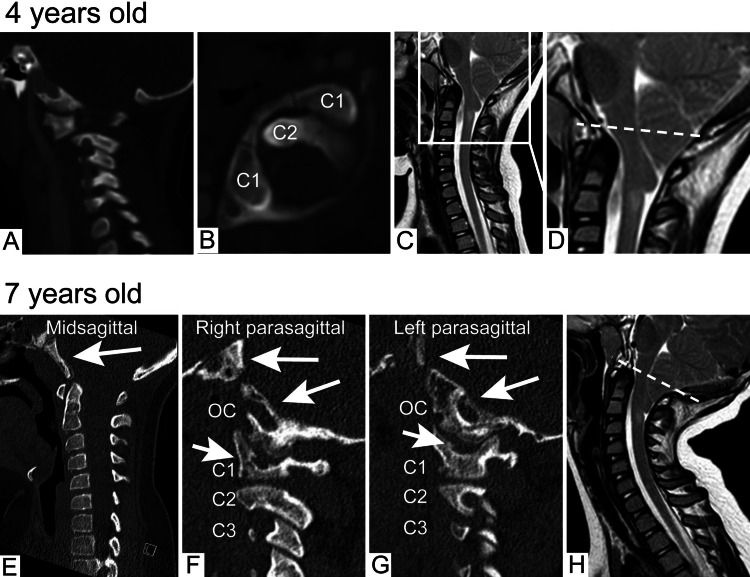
Follow-up imaging when the patient was 10 years old. Midsagittal (A) and parasagittal (B and C) CT demonstrating progressive osteolytic changes in the clivus, OC, and C1 (arrows) now with fusion of the OC to C1. Sagittal MRI (D) of the cervical spine with gadolinium contrast demonstrating worsening of caudal tonsillar descent and progressive bone marrow changes with contrast enhancement involving the CVJ and C1-C4 vertebral bodies (arrow). Note the McRae line (dashed line). Radiograph (E) and bone-window CT images (F) of the skull and skull base (G) demonstrating extensive osteolytic changes of the calvaria, facial bones, and skull. The overall radiological findings were considered diagnostic for GSD. Image reproduced from Syed et al. (2025) [8] under the CC BY-NC-ND 4.0 license. CVJ = craniovertebral junction; GSD = Gorham-Stout disease
